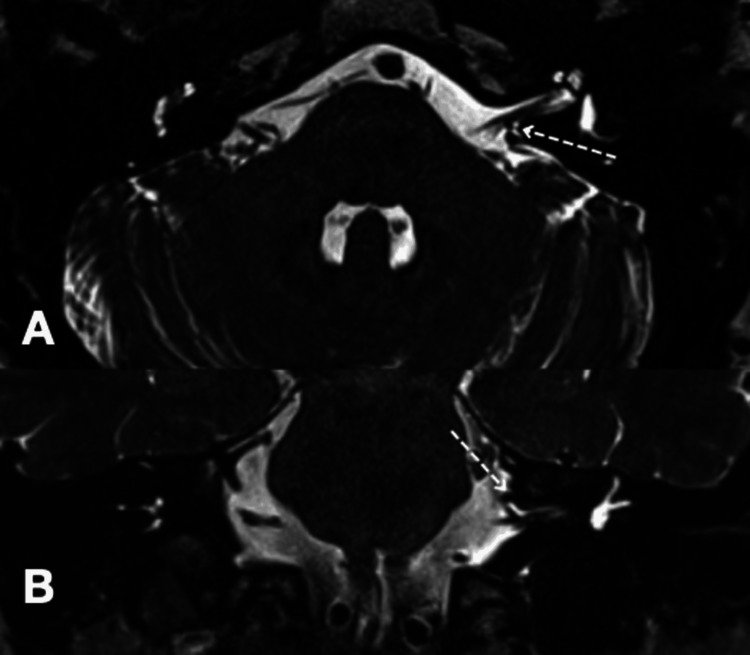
Magnetic resonance image analysis of Case 4 of constructive interference in steady state (CISS) sequence (axial A and coronal B) showing a vascular loop in the left cerebellopontine angle causing mild compression over the seventh and eighth cranial nerve complex along the posterosuperior aspect (dotted arrow).
